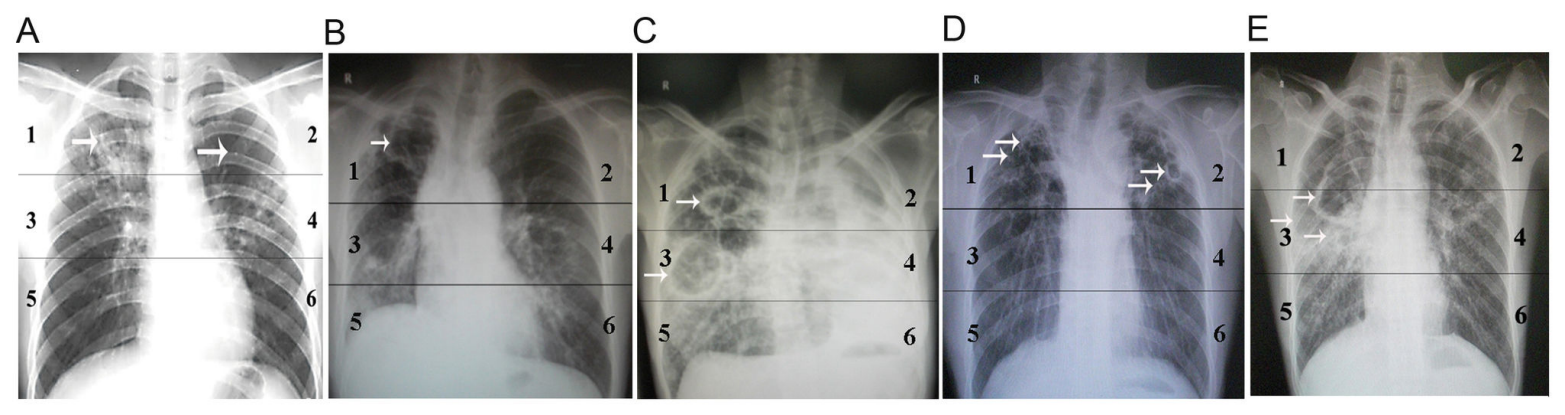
X-Ray images for lesion severity scoring. The white arrows indicate the lesions and cavities. A: Field 1, ≥50% of area affected = score of 2; Field 2, <50% of area affected = score of 1, B: Field 1, single cavity, <2cm diameter = score of 0.25, C: Field 1, single cavity, 2-4cm diameter = score of 0.5; Field 3, single cavity, >4cm diameter = score of 1, D: Field 1, multiple cavities, largest <2cm diameter = score of 0.5; Field 2, multiple cavities, largest 2-4cm diameter = score of 1, E: Field 3, multiple cavities, largest >4cm diameter = score of 2.
